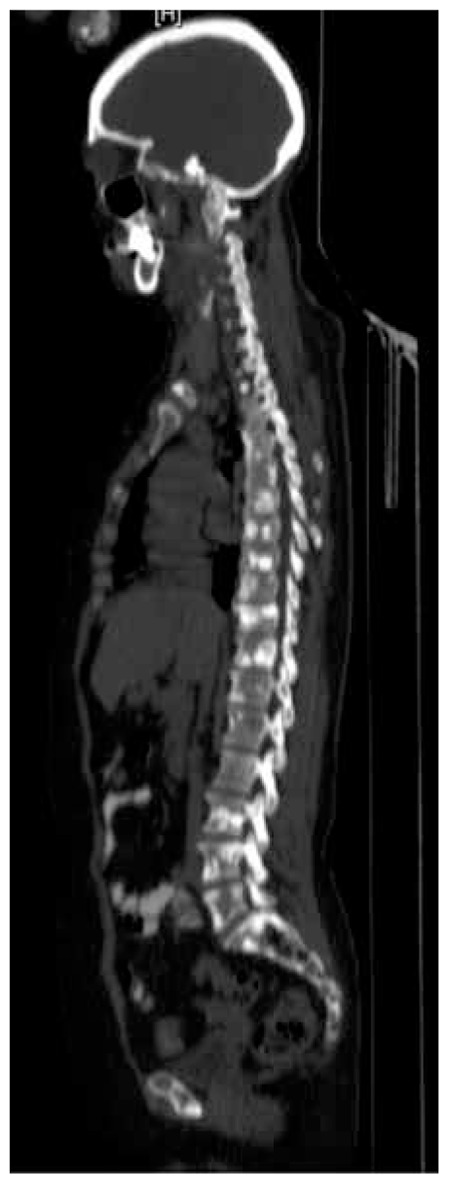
Sagittal computed tomography image of the patient. Multiple sclerotic lesions were seen in the entire skeleton
